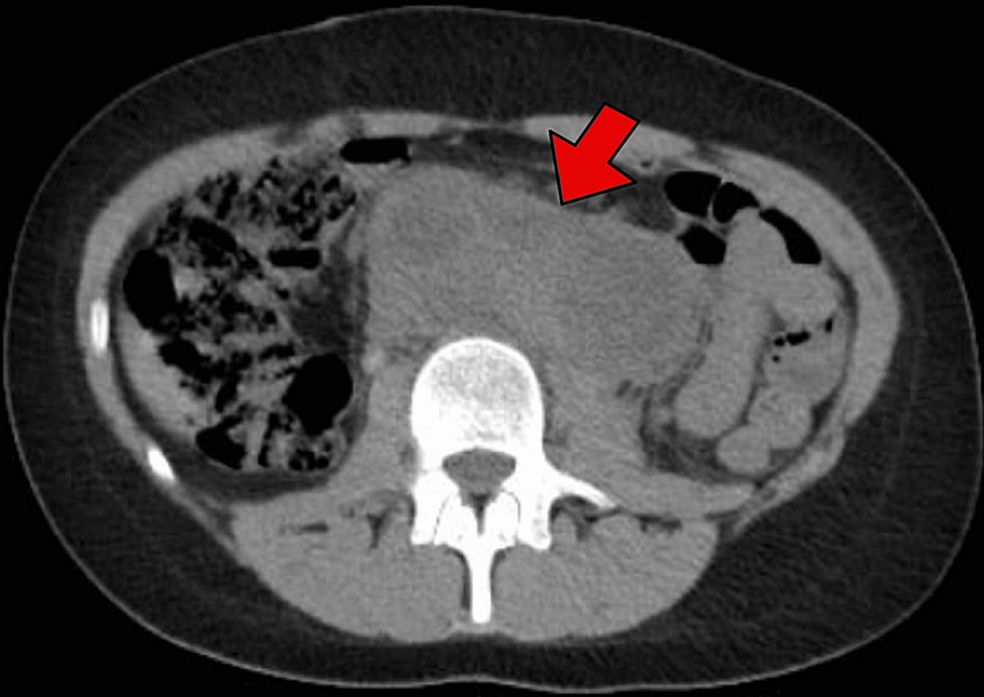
Computed tomography scan of the abdomen The exam revealed a retroperitoneal expansive lesion located in the epi/mesogastrium. The lesion was lobulated, predominantly hyperdense with intervening hypodense areas (arrow). It measured 12.0 x 9.8 x 4.7 cm and surrounded the aorta and the inferior vena cava (not shown in the image). The findings were suggestive of secondary retroperitoneal tumoral lesion.
